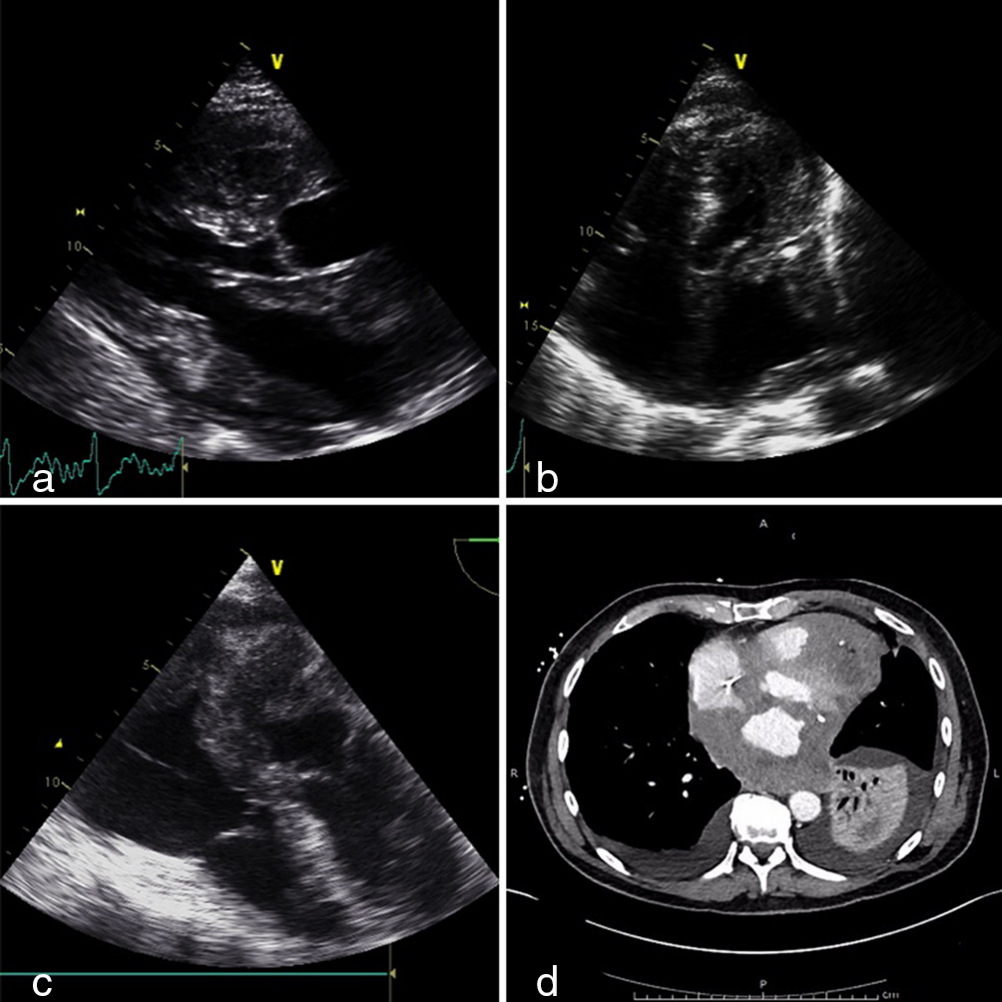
(a) Transthoracic echocardiogram (parasternal long axis view) showing marked infiltration of the left atrium and both the ventricles by diffuse large B-cell lymphoma. (b) Transthoracic echocardiogram (apical four-chamber view) showing marked infiltration of the left atrium and both the ventricles by the diffuse large B-cell lymphoma. (c) Transoesophageal echocardiogram (three-chamber view) showing extensive infiltration of the left atrium and the ventricles by diffuse large B-cell lymphoma. (d) CT imaging of the thorax showing extensive lymphoma infiltration of the heart.
